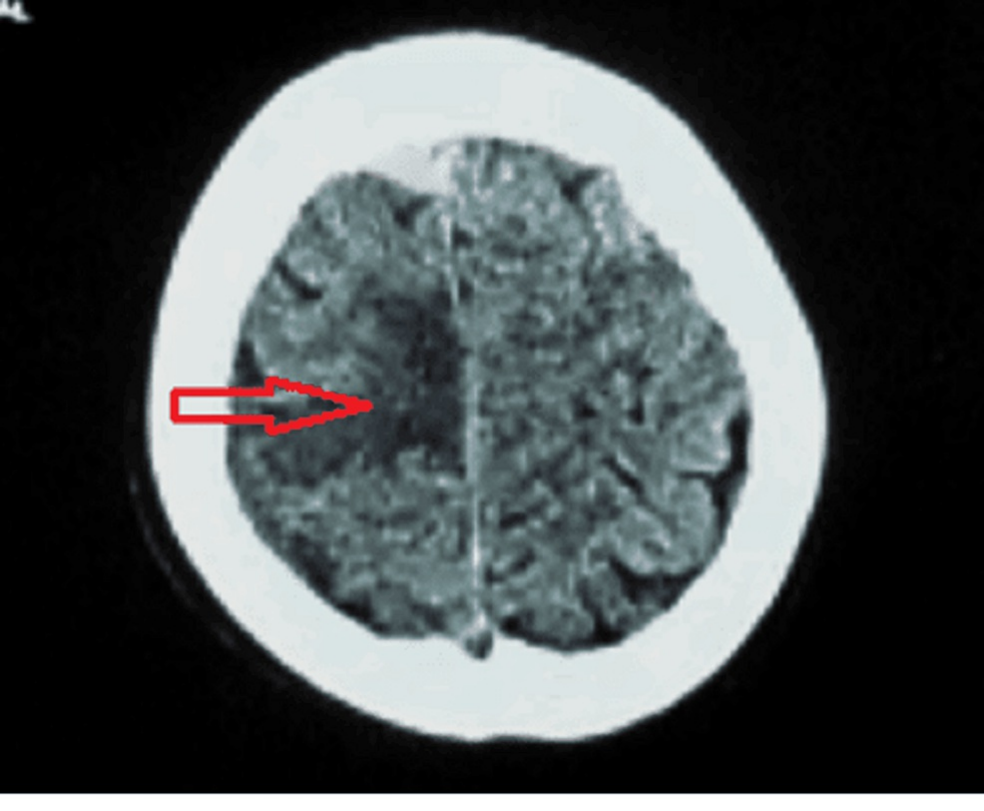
Non-Contrast CT-Scan Non-contrast CT brain showing hypodensity in the frontoparietal area
